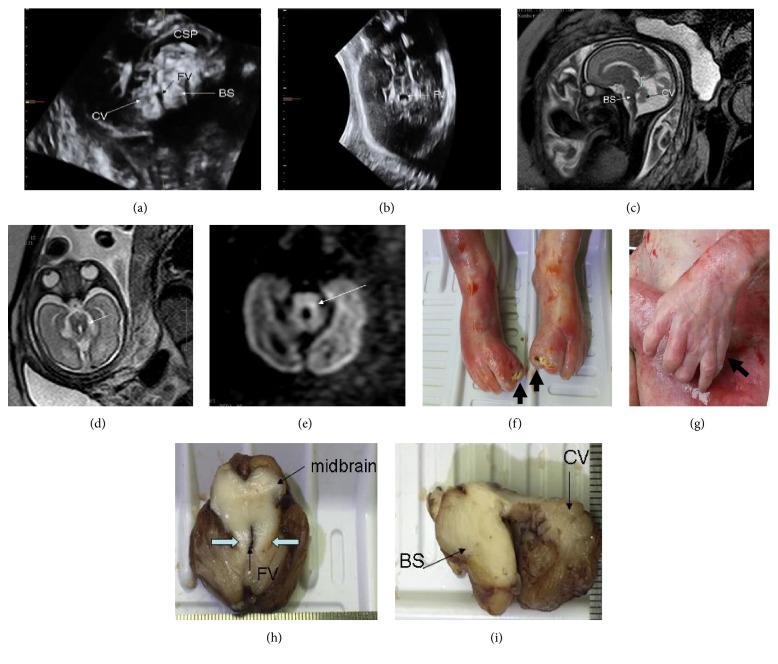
Clinical features of the fetus. (a) The sagittal ultrasound image shows hypoplasia of the cerebellar vermis and a triangular shaped fourth ventricle. (b) The axial ultrasound image shows a 'bat-wing'-shaped superior fourth ventricle. (c) The sagittal MRI image shows vermian agenesis and hypoplastic superior cerebellar peduncle. The axial T2-weighted (d) and diffusion-weighted (e) MRI images show prominent interpeduncular fossa and a deep cleft between thickened cerebellar peduncles comprising the molar tooth sign, as indicated by the arrows. After artificial abortion, polydactyly of both feet (f) and left hand (g) was noted, and the brain autopsy revealed the molar tooth sign (h, i). CV: cerebellar vermis, BS: brainstem, FV: fourth ventricle, CSP: cavum septi pellucidi.
